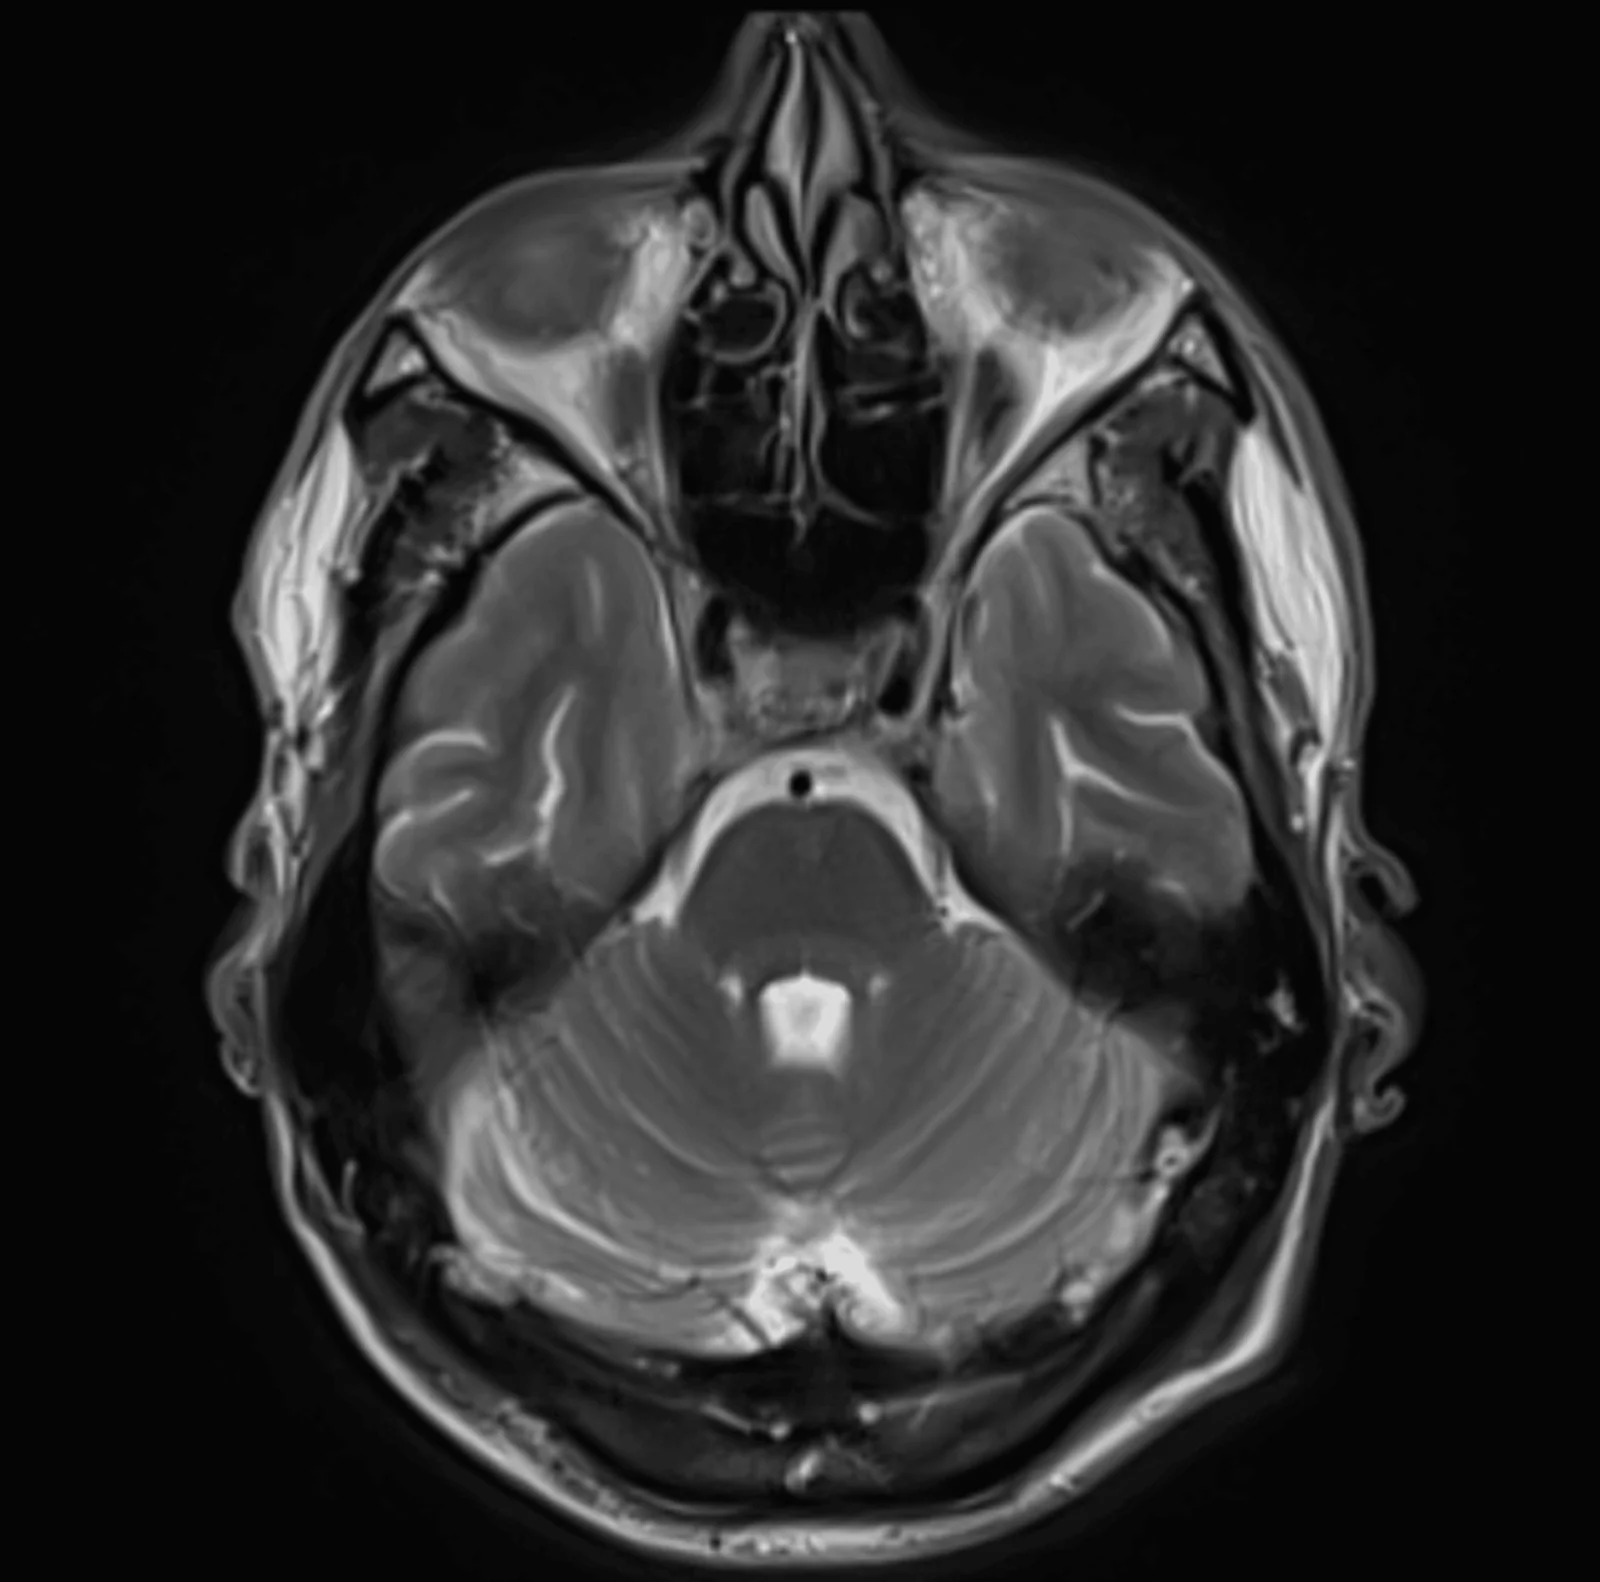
Axial T2 MRI of brain Negative for any space-occupying lesions, focal parenchymal abnormalities, or other structural CNS abnormalities. The MRI was obtained as part of the broader neurologic evaluation rather than specifically for evaluation of the patient's episodic weakness.
